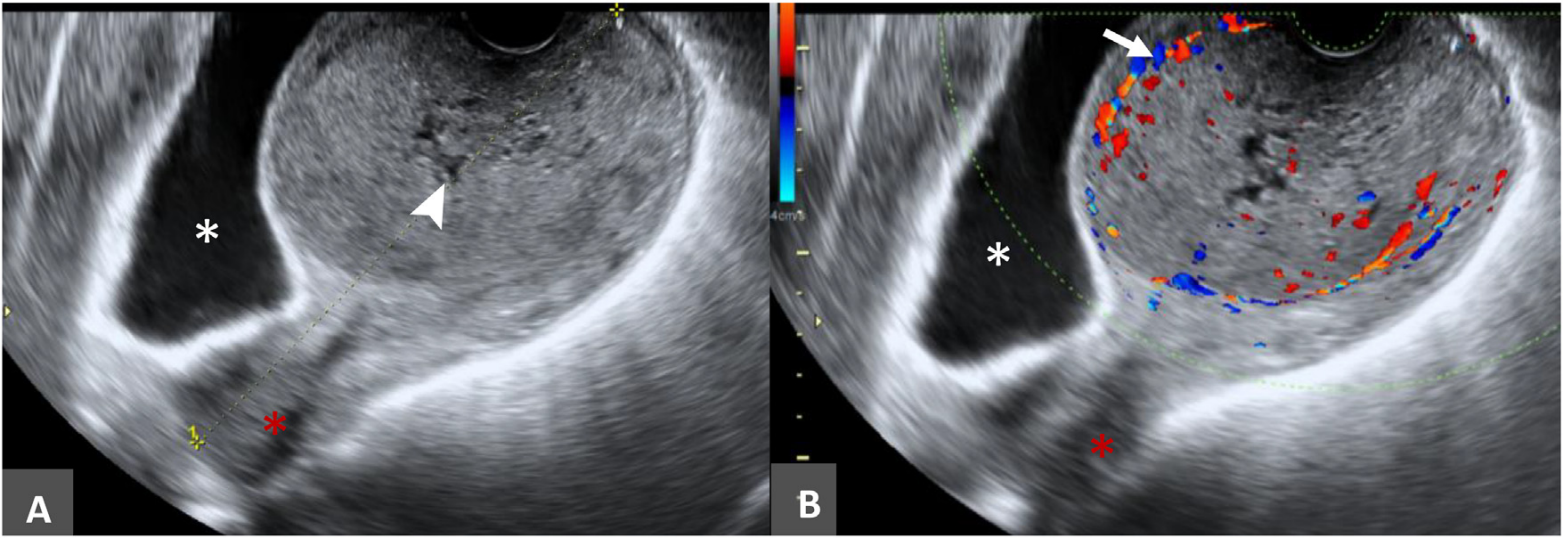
Initial imaging at presentation. (A) Transvaginal grayscale ultrasound: well-circumscribed, heterogeneous mass within the cervical canal with internal cystic spaces. (B) Color Doppler: peripheral vascularity surrounding the lesion.
